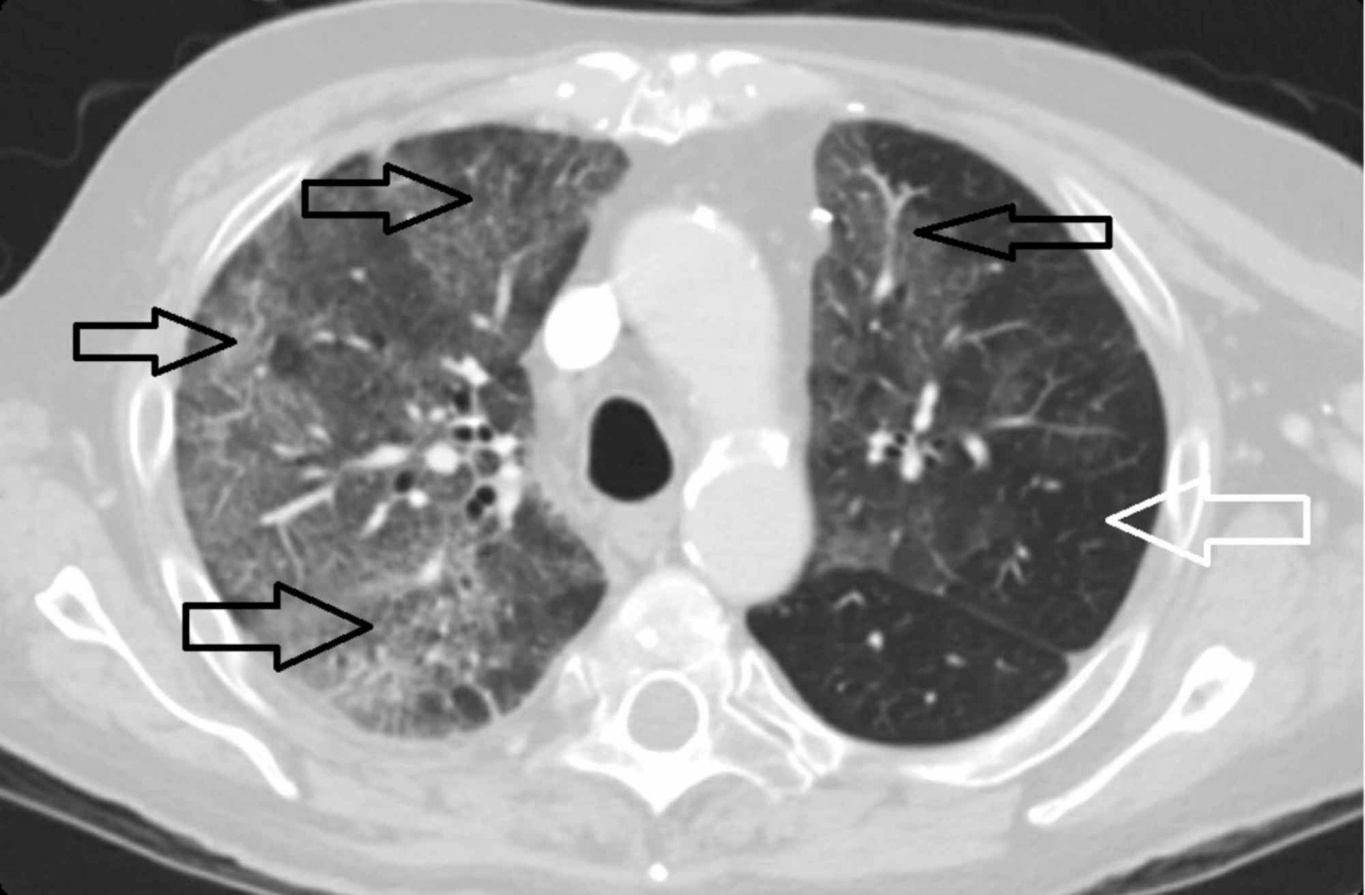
Cross section view of CT scan of thorax demonstrating bilateral right-sided predominant multi-focal parenchymal lung disease in the form of ground-glass opacities (black arrows). Left lung is less affected and shows areas of relatively preserved parenchyma (white arrow).
